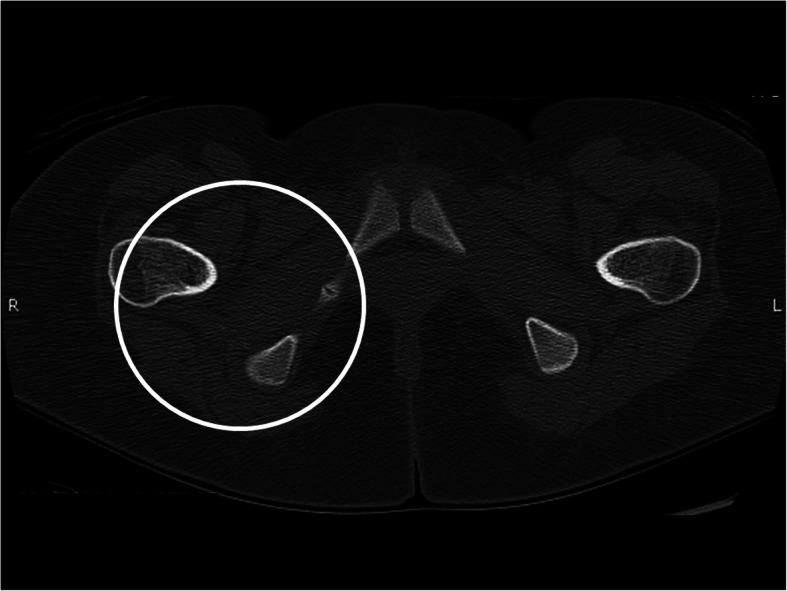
Axial CT image of the pelvis from a follow-up examination showed complete resolution of the area of bulky calcification (white circle)
